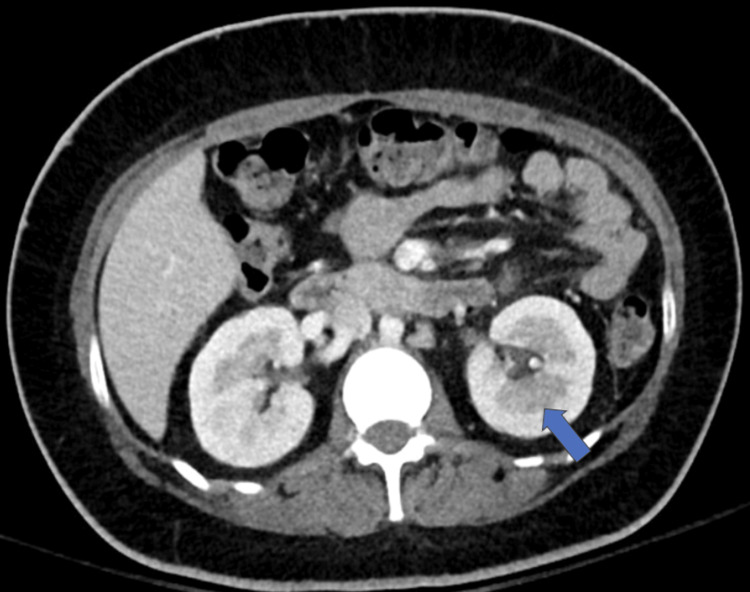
Contrast-enhanced CT of the abdomen Contrast-enhanced CT of the abdomen demonstrating a focal hypodense region within the left renal parenchyma (blue arrow), consistent with lobar nephronia (acute focal bacterial nephritis), and measuring approximately 3.6 cm. There is no rim enhancement or fluid collection, arguing against a renal abscess. Additional subtle inflammatory changes are noted in the contralateral kidney, suggestive of early right-sided pyelonephritis CT: computed tomography
